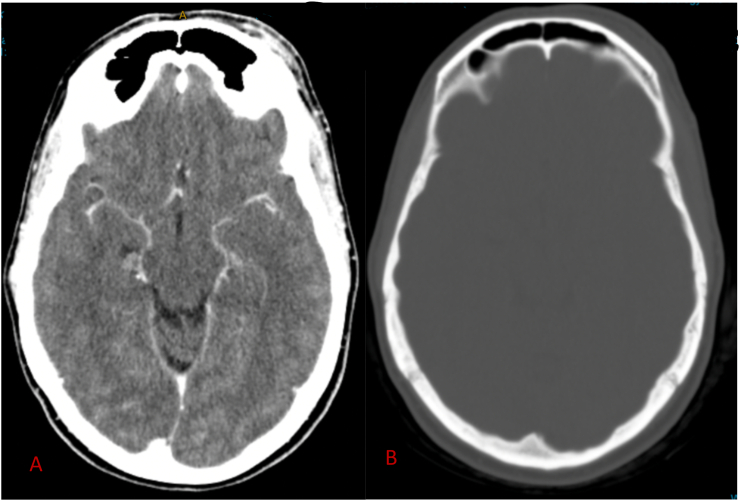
(A and B): A Computer Tomography (CT) scan with pre and post contrast that was performed 10 days post-surgery. Fig. 2A is an axial CT bone window at the level on the frontal sinuses showing no osseous involvement or destruction. Fig. 2B shows post contrast enhancement at the left supraorbital subcutaneous area, which could either be secondary to post-surgical changes or residual PEM. There is no pre operative CT or MRI to compare to.
